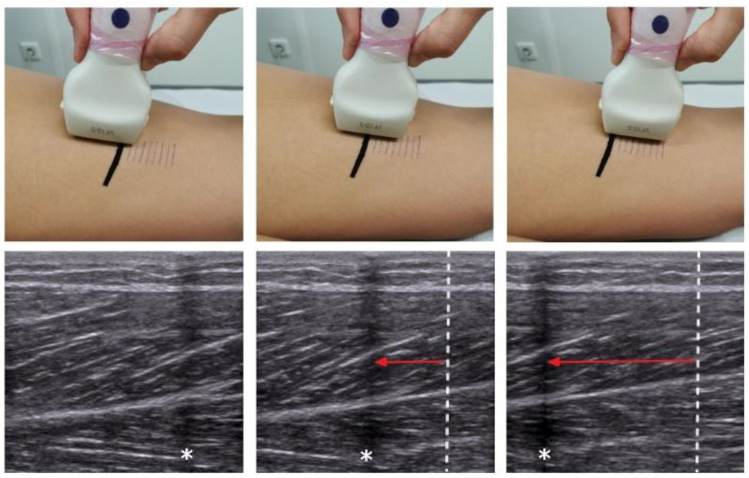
Position and movement of the ultrasound (US) transducer on the skin (top) and the resulting US images (bottom). Initially (left), the shadow of the reflective tape (asterisk) can be seen at the right border. Upon probe movement (middle and right), this shadow is displaced relative to the original starting position (dotted line). The distance (red arrow) is quantified using software.
